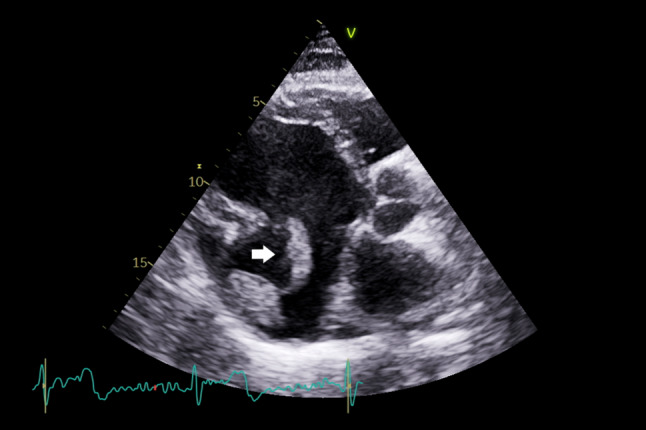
Transthoracic echocardiogram parasternal long-axis view with a posterior tilt showing a fragmented and mobile thrombus (white arrow) within an inferior wall aneurysm
